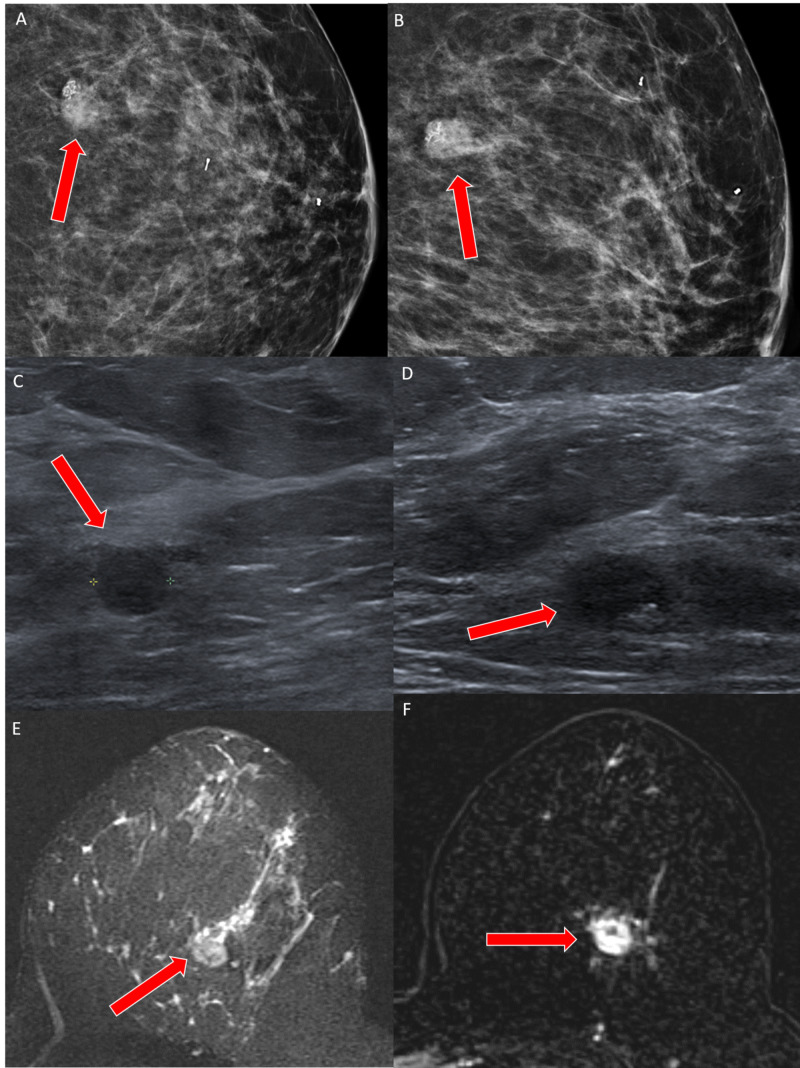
Case 3 imaging findings A, B: Craniocaudal and mediolateral oblique tomosynthesis views of the left breast show a new, round, equal density mass (red arrows) with partially obscured margins and coarse calcifications. C, D: Ultrasound demonstrates a circumscribed round hypoechoic mass (red arrows) with echogenic calcifications and partially obscured and microlobulated margins. E, F: MRI shows a round, T2, hyperintense, homogeneously enhancing mass (red arrows). MRI: magnetic resonance imaging
